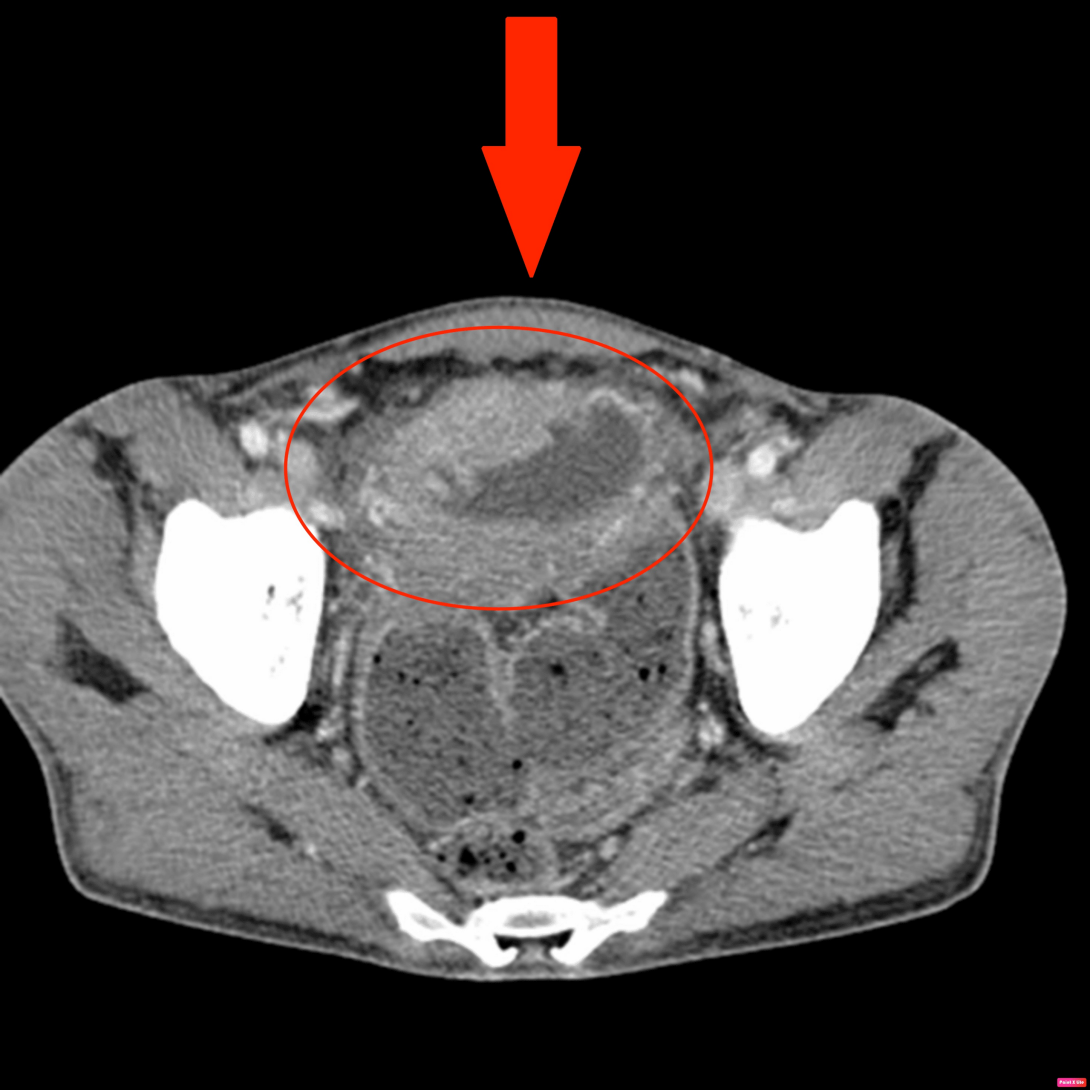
Axial computed tomography showing marked thickening and inflammation of the bladder wall.
